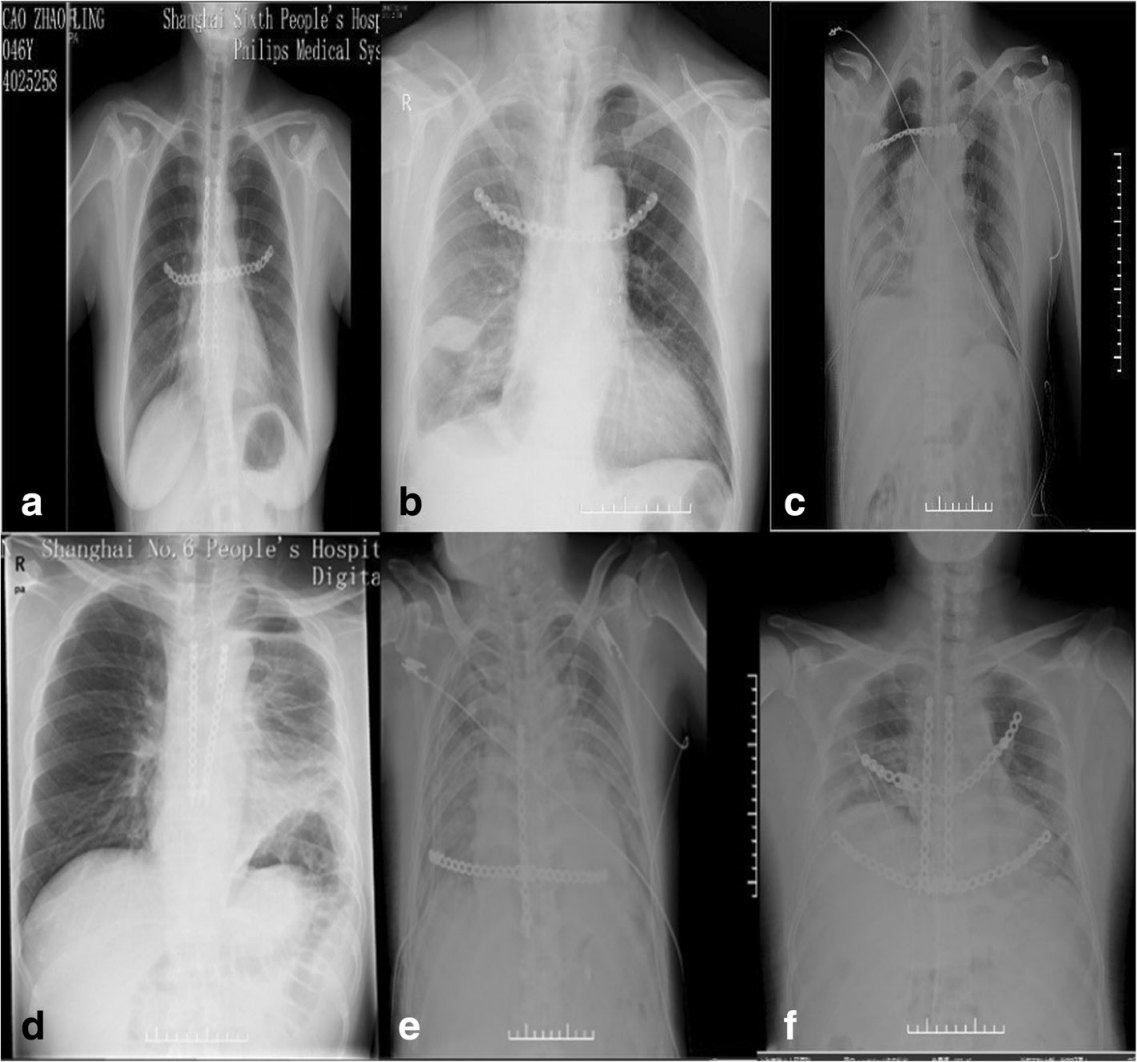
Postoperative X-ray films of the case series. (a is for Case 1, b is for Case 2, c is for Case 3, d is for Case 4, e is for Case 5, f is for Case 6)
